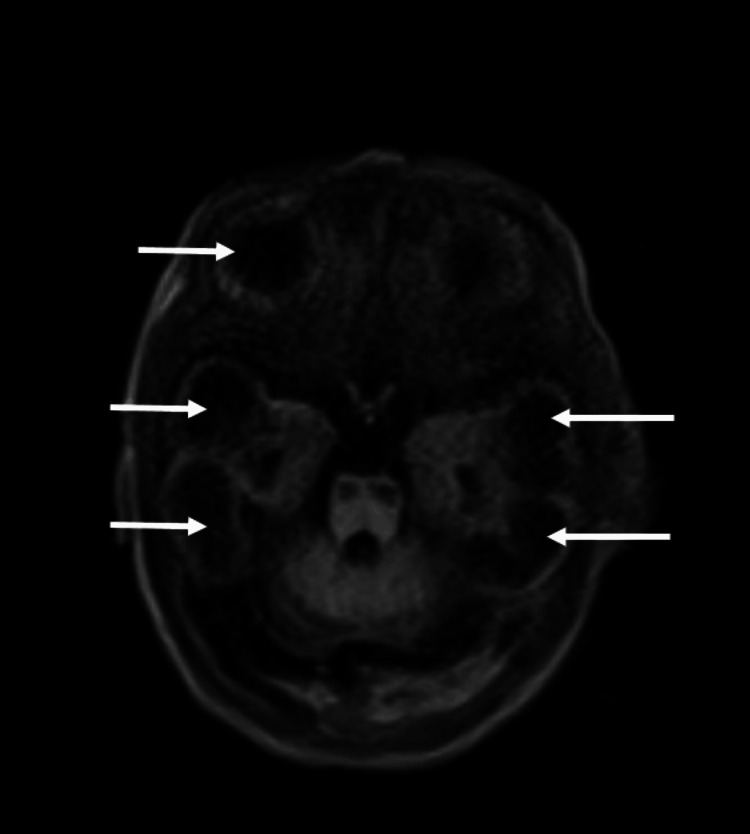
MRI brain MRI Brain (T2-axial image) showing cerebral atrophy, and multi-cystic encephalomalacia (white arrows) with cystic changes in the midbrain.
